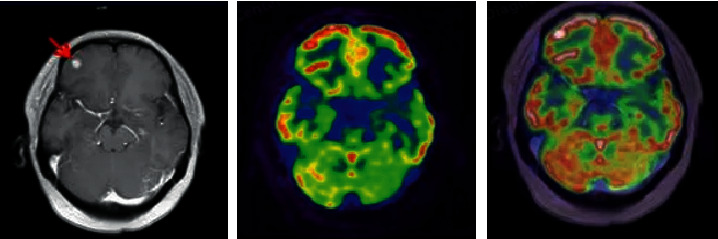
PET + MRI fusion images of glioma patients. From left to right, they were the fusion images and the images at 8 h and 24 h after the tracer injection. The arrows indicate the lesions.
